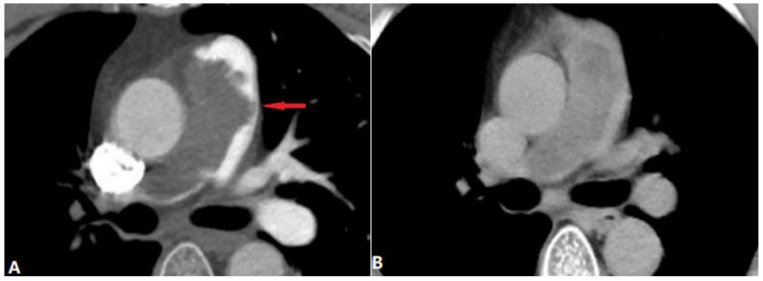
Pulmonary artery sarcoma with extensive involvement of the main pulmonary artery and the right trunk. (A): Contrast-enhanced CT showing a soft tissue mass filling the main and left pulmonary arteries (arrow). (B): The filling defects show delayed enhancement.
